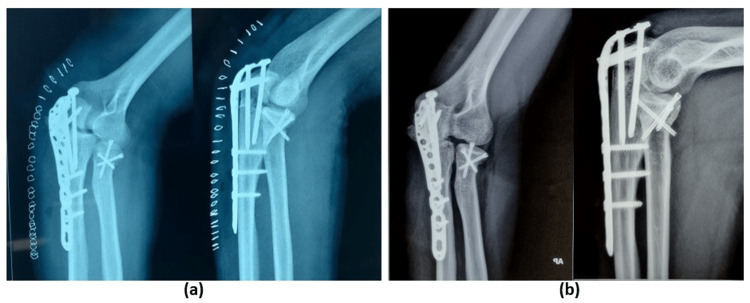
(a) Immediate postoperative radiograph depicting a contoured olecranon plate fixation and radial head fixation with headless cancellous screws (tripod fixation). (b) Twelve-month follow-up with a complete union of fractures.
